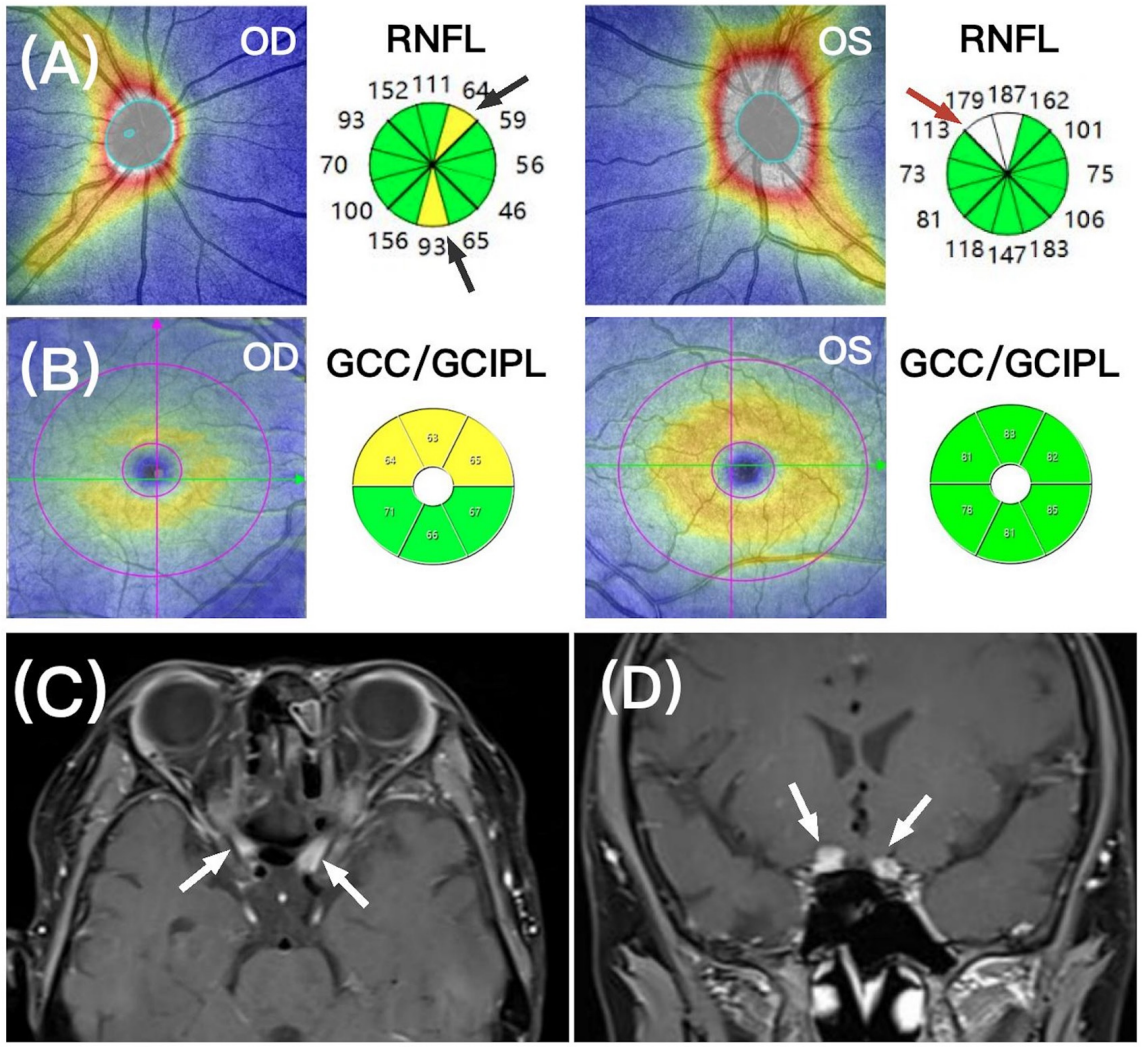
(A) Recurrence at 1 year after treatment, OCT-RNFL (top panel) showed focal mild thinning (black arrows, sectors in yellow color) of the right optic nerve, and mild edema in the left optic disc (red arrows, sectors in white color). (B) OCT-GCC (middle panel) showed superior thinning of the right eye, indicating partial optic atrophy. The left eye showed no GCC/GCIPL thinning. (C,D) Orbital MRI showed bilateral prechiasmatic optic nerve thickening and enhancement (white arrows).
